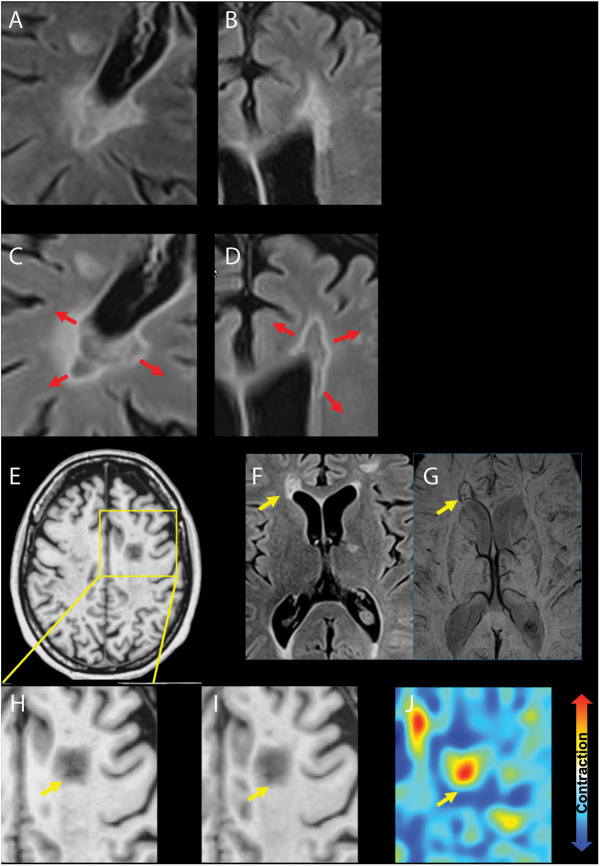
Examples of Slowly Expanding Lesions and a Paramagnetic Rim Lesion Axial FLAIR images showing slowly expanding lesions in the periventricular regions developing between baseline (A and B) and 2-year follow-up (C and D). The red arrows indicate the direction of the lesion expansion. Note also the increase in hypointensity indicating progressive tissue destruction. Axial FLAIR image (F) and SWI (G) showing a paramagnetic rim lesion (yellow arrow). (E) Axial T1-weighted image showing an hypointense lesion in the corona radiata at baseline (H) (yellow arrow) and 2 years follow-up (I) (yellow arrow), with corresponding deformation map (L), which shows in red a concentric expansion of the lesion over time (called slowly expanding lesion) (positive Jacobian >0) and in blue a shrinkage (Jacobian ≤0). FLAIR = fluid-attenuated inversion recovery; SWI = susceptibility-weighted imaging.
